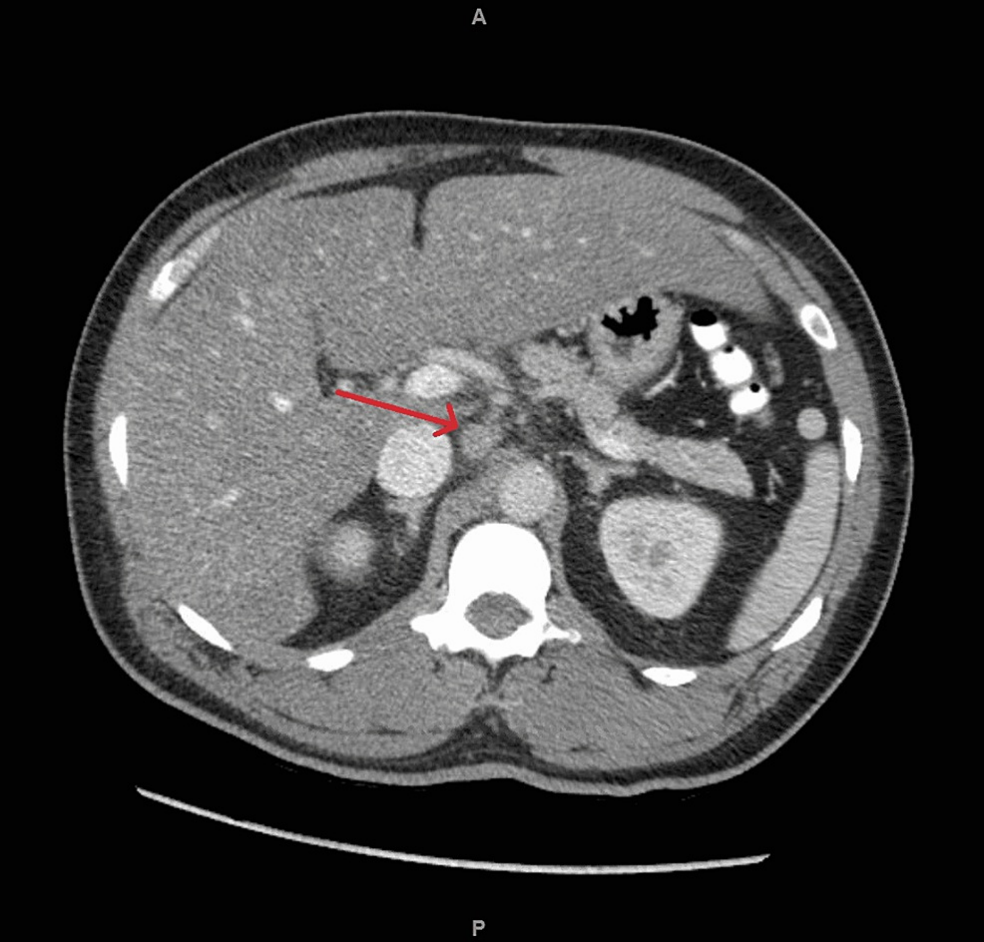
Computed tomography of abdomen and pelvis showing enlarged lymph nodes (red arrow) in upper retroperitoneum and gastric hepatic ligament
